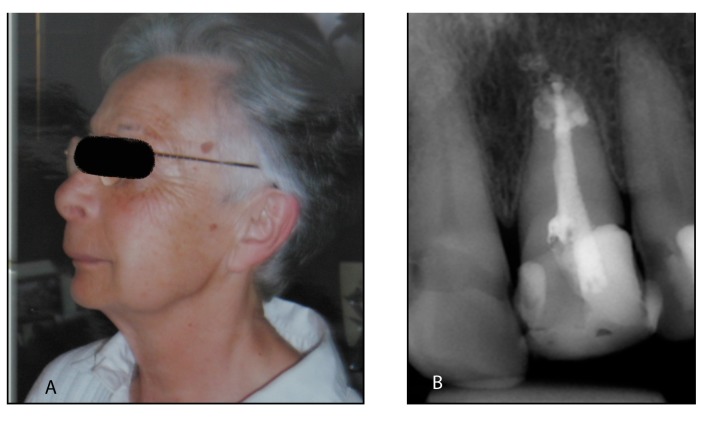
Case report nº1. (A) Lateral view of the patient 6 days after sodium hypochlorite extrusion of the left upper central incisor. (B) Periapical radiography of the affected incisor with periapical radiopaque areas, 1 year after sodium hypochlorite extrusion.
